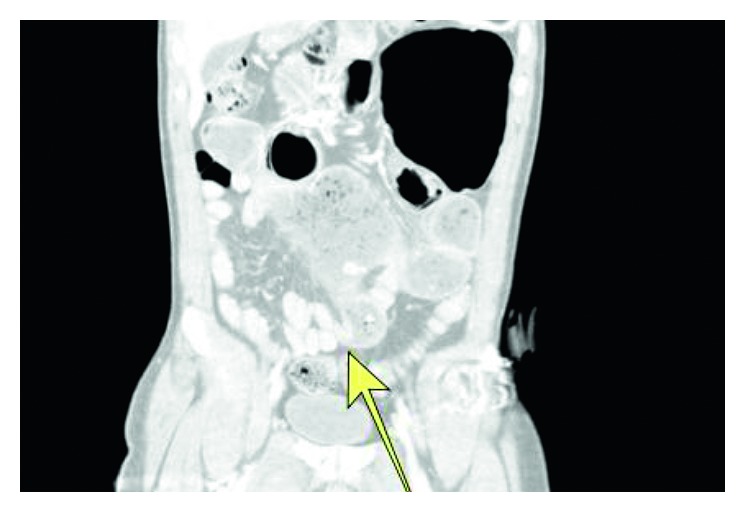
Computed tomography image demonstrating the small bowel obstruction secondary to the phytobezoar. A transition point is seen in the midline lower abdomen in the region of the proximal ileum, with distended proximal small bowel loops containing fecalized material and decompressed distal small bowel loops (yellow arrow). There is a similar appearance of the distended left upper quadrant bowel anastomosis site, as well as within the side-to-side small bowel anastomosis in the midabdomen. A small amount of mesenteric fluid is seen, without significant ascites, small bowel wall thickening, differential mucosal enhancement, or pneumatosis to indicate bowel compromise.
